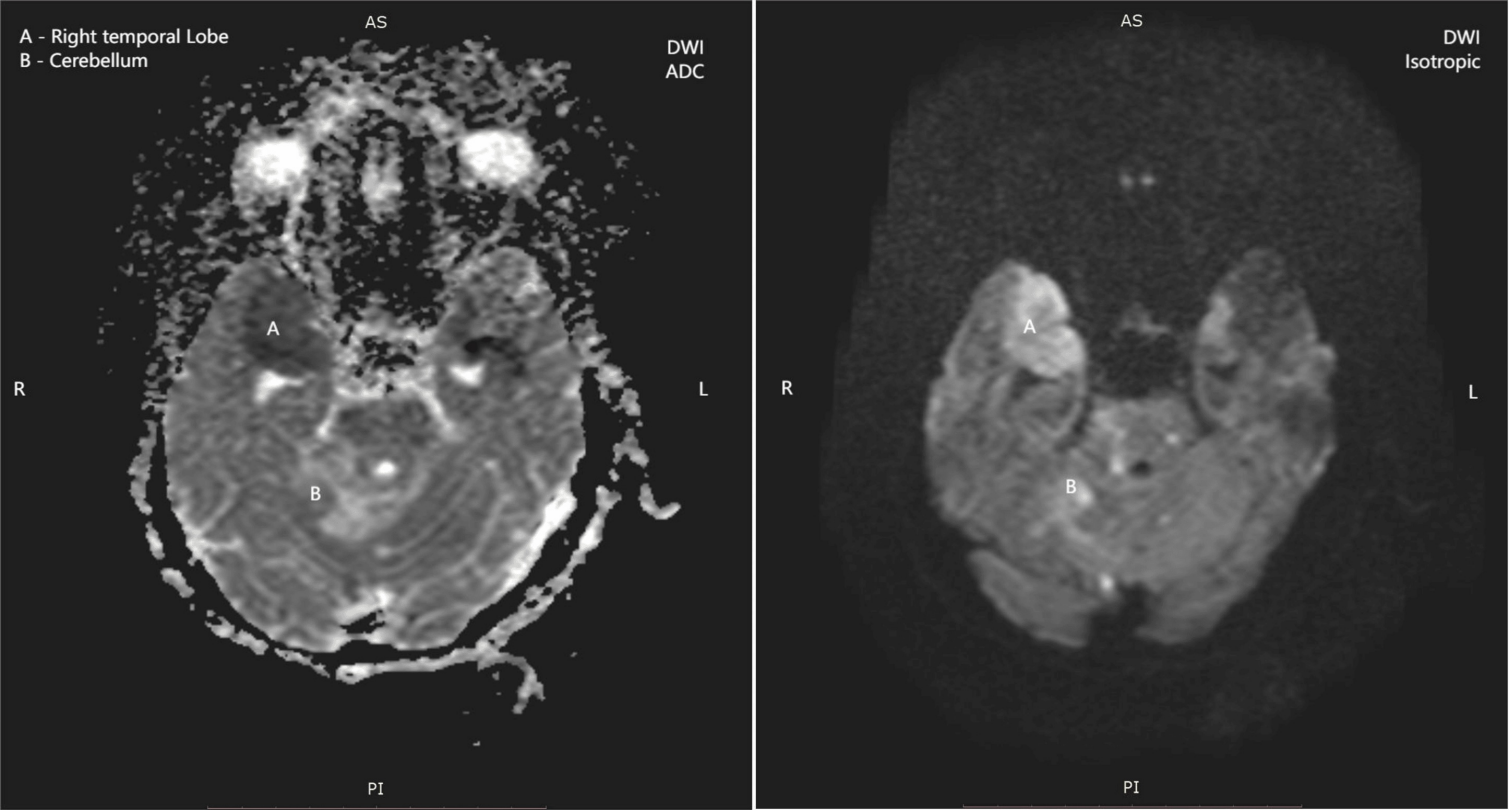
Axial DWI and corresponding ADC map showing diffusion restriction in the right temporal white matter and cerebellar hemisphere ADC, Apparent diffusion coefficient; DWI, Diffusion-weighted imaging
